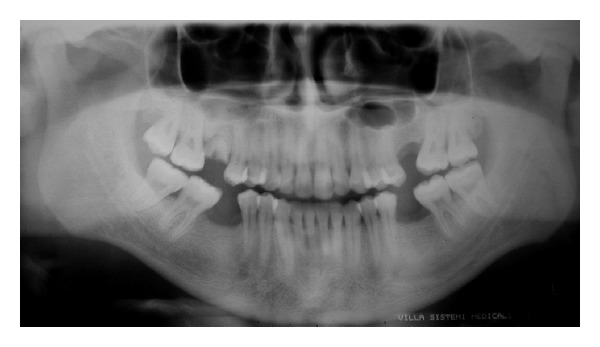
Panoramic radiograph showing radiolucent area surrounded by radiopaque halo located in the periapical region of the upper first and second premolars.
